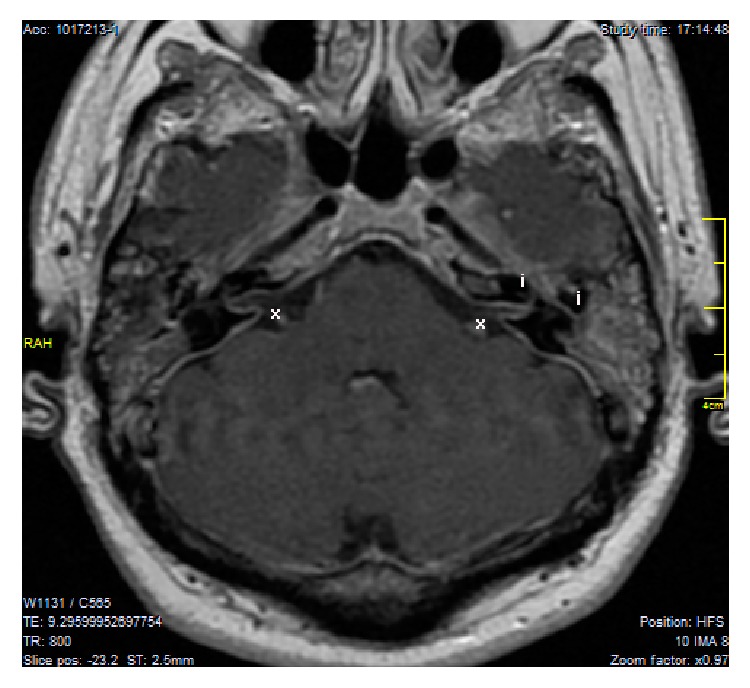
T1-weighted Gd-MRI, axial image*. x: symmetrical lineal diffuse thickening and enhancement of the dural layer of the IAC. i: left labyrinthine and tympanic facial nerve enhancement.
